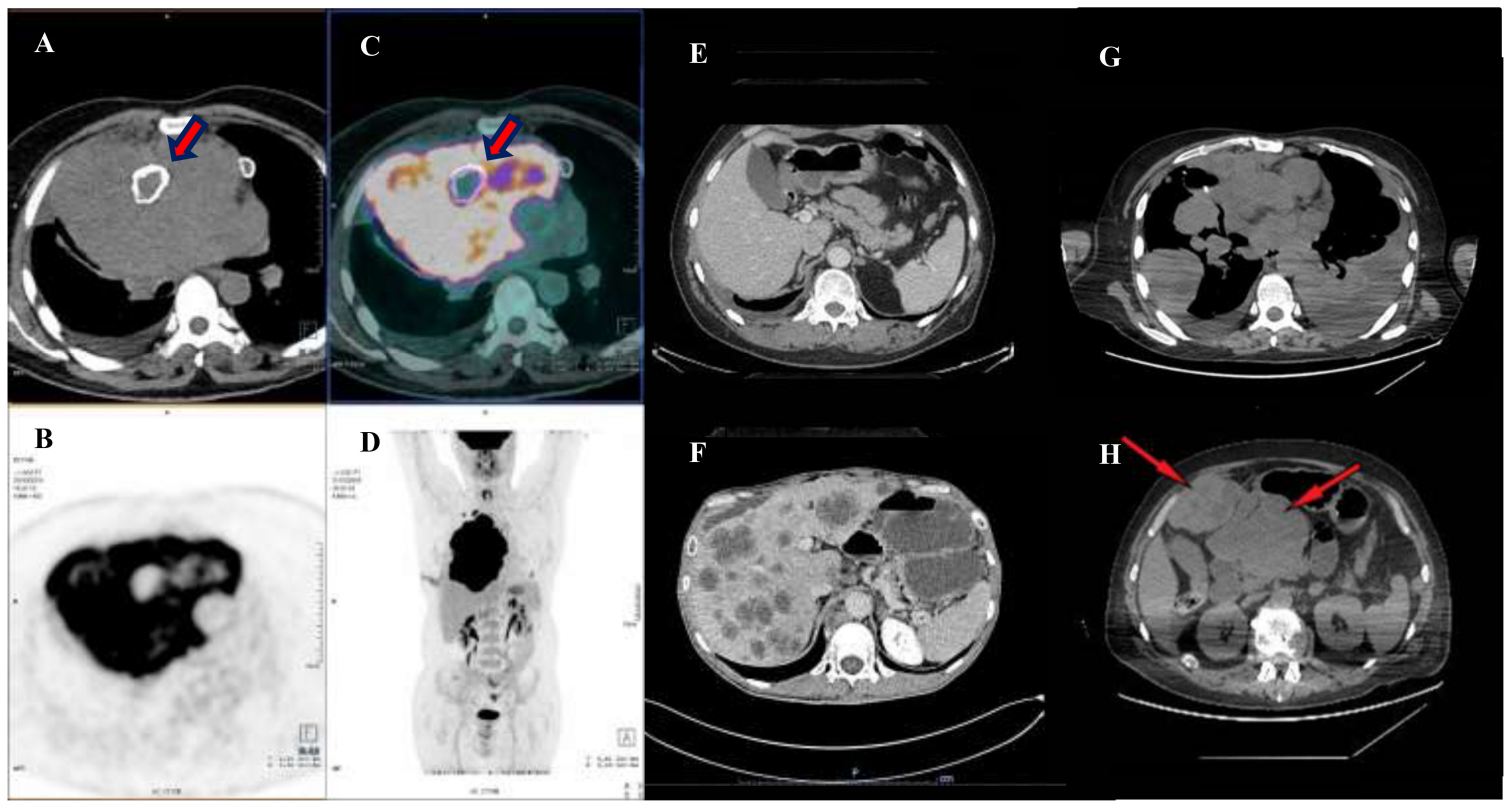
Preoperative and pre-chemotherapy PET-CT scan. (A–D) shows a PET-CT scan performed in October 2019, evidencing a bulky expansive lesion occupying the large part of mediastinum and dislocating the right lung peripherally. Note thick red arrows pointing to a calcified necrotic center, englobed in the neoplatic mass. Thereafter, an induction chemotherapy was performed with the aim of reducing the mass and leading the patient to the surgical excision. Image in (E) shows a post-surgical CT scan performed in April 2020, during outpatient clinic follow up, note that the abdomen window was free from disease. However, an early relapse occurred in August 2020 with development of hepatic metastases (F). A first line of therapy SMT-oriented, with a sequential use of Nivolumab and Ipilimumab was used. However, after four months of therapy, a restaging with a CT scan was performed highlighting a progressive disease. The disease spread in the lung bilaterally (G) and in the abdomen (H) with a disease extension of the II and III hepatic segments over the epigastrium (thin red arrows).
